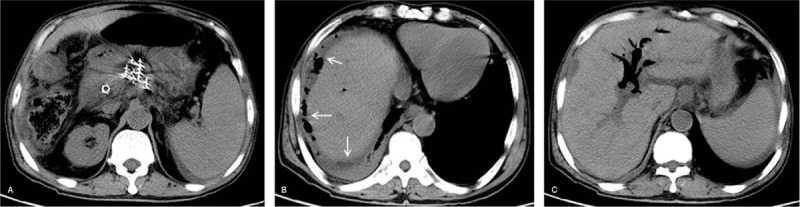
Intestinal perforation, abdominal cavity infection and biliary pneumatosis were complicated in an advanced pancreatic cancer patient, who was treated by iodine-125 seed implantation. (Left) Iodine-125 seeds were implanted under CT-guide. (Center) Abdominal cavity infection resulted from intestinal perforation was revealed (arrow) when CT follow-up was performed on the 7th day after implantation. (Right) Biliary pneumatosis were shown on CT scans.
